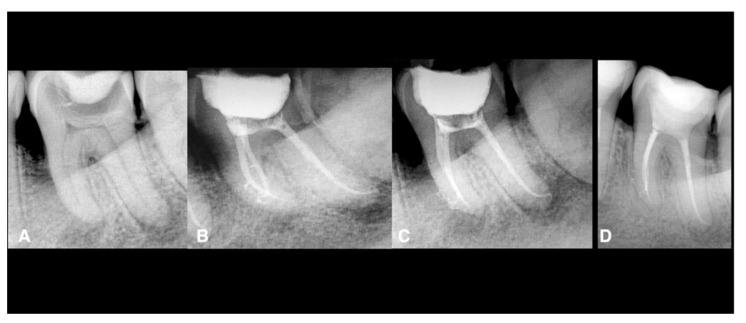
Endodontic treatment of a second mandibular left molar. The tooth was necrotically associated with periapical lesions. The treatment was done using conservative shaping and the 3D cleaning procedure, as mentioned in the Materials and Methods. (A) Preoperative X-ray, (B) postoperative X-ray, (C) postoperative X-ray with different angulation, (D) 2-year follow-up showing healing.
